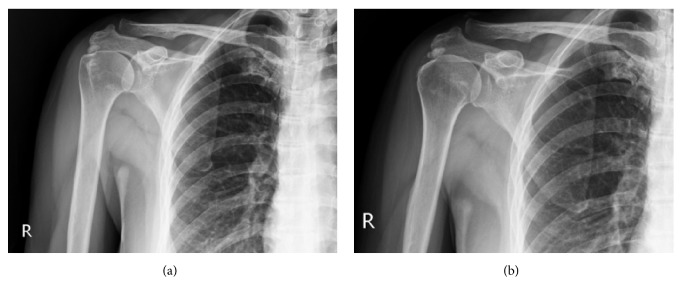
Application of ESWT on type I calcific shoulder tendinosis. (a) Plain film before ESWT (b) 6 months after ESWT. No resorption of calcium deposits without interval change regarding CMS and VAS.
